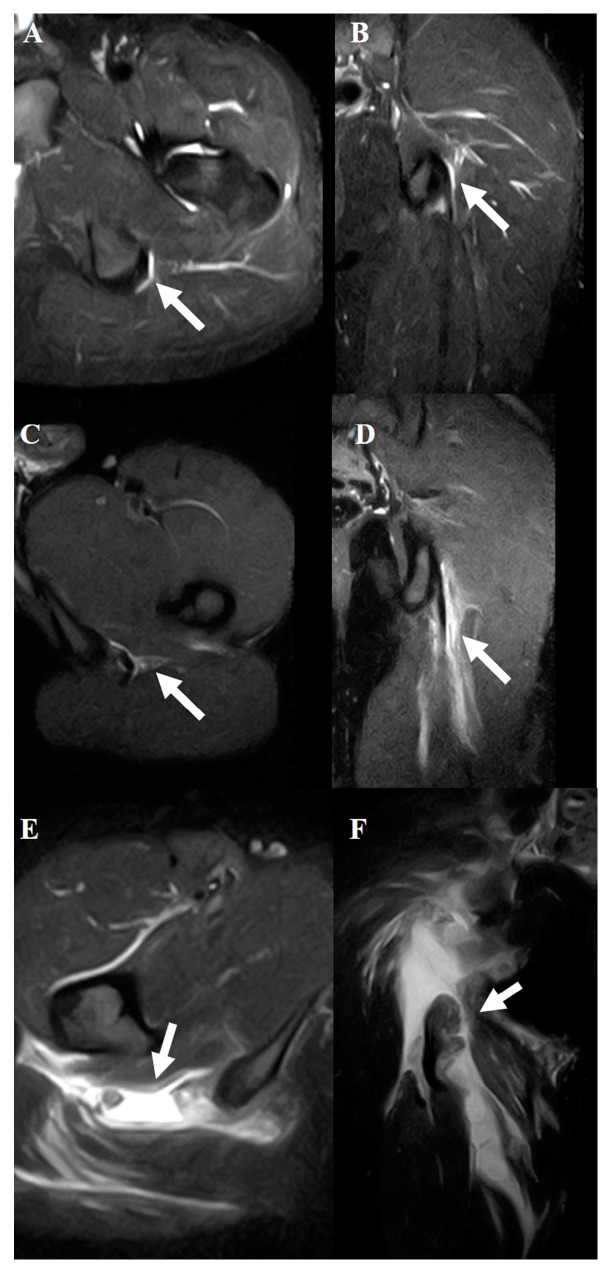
Typical MRI findings in acute hamstring strains. All views show a tendon injury of the semimembranosus muscle. Axial (A) and coronal (B) views of grade 1. Axial (C) and coronal (D) views of grade 2. Axial (E) and coronal (F) views of grade 3. The white arrows indicate injured areas.
